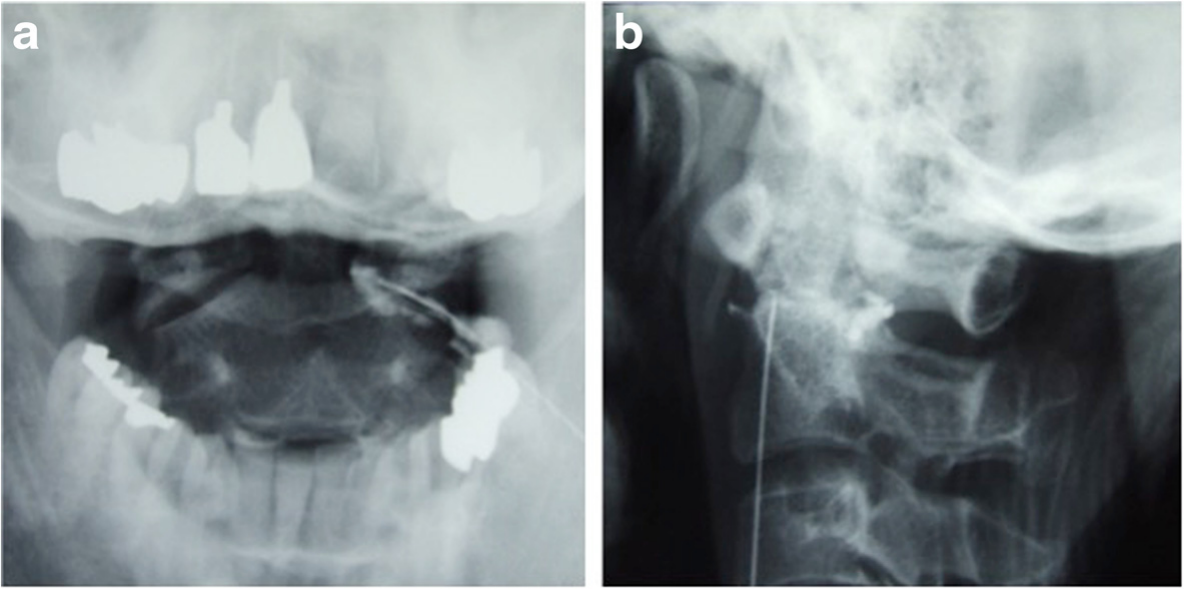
Anterior-posterior (a) and lateral (b) radiography after radiopaque contrast is injected to lateral atlantoaxial joint. Radiopaque contrast did not go around the dens
